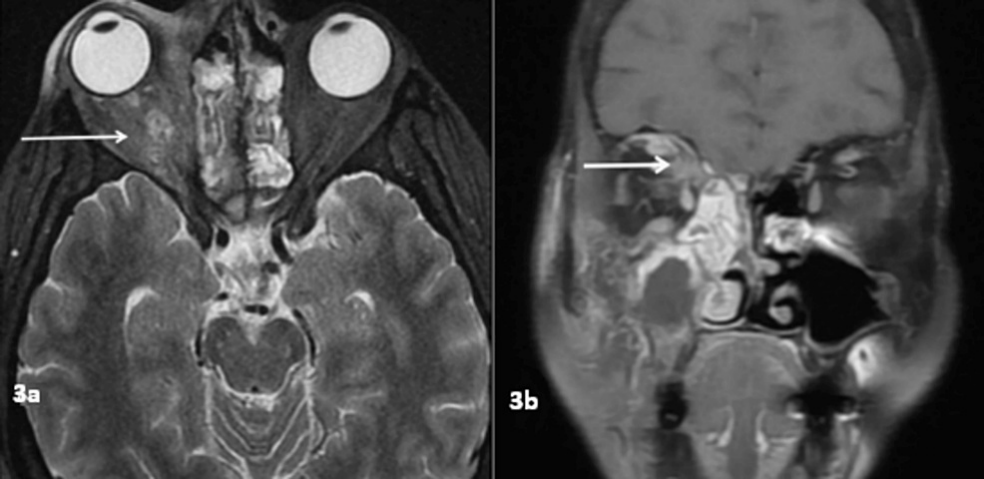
Orbital involvement 3a: Coronal T2WI of a 43-year-old patient with ROCM showing altered signal intensity in the right intra- and extraconal compartment (arrow). 3b: Coronal post-contrast FS T1WI showing abnormal enhancement of the right intra- and extraconal fat (arrow). T2WI: T2-weighted image, ROCM: rhino-oculo-cerebral mucormycosis, FS T1WI: fat-suppressed T1-weighted image
